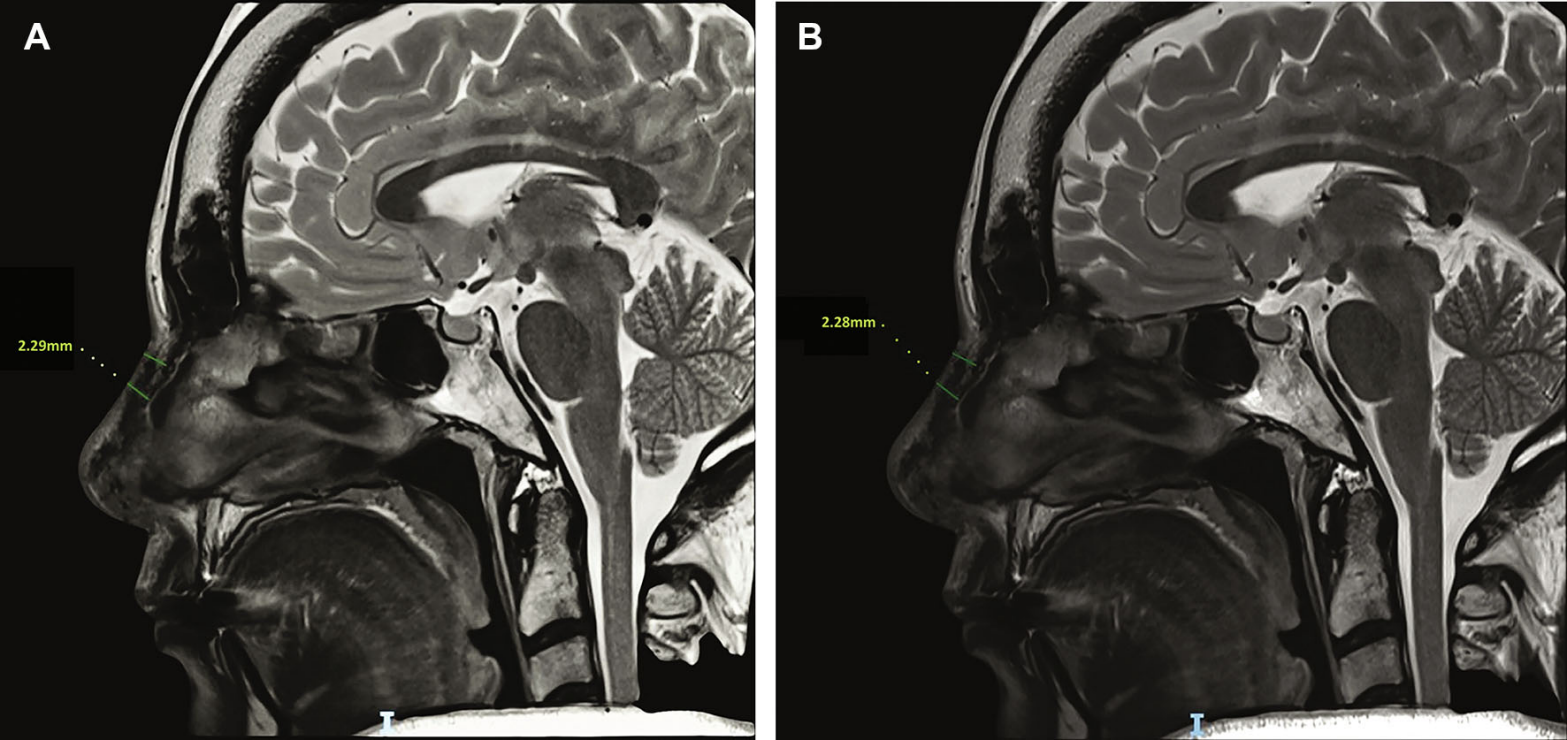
Example of a 2-week postoperative MRI a. The fascia construct maintains its volume and shape throughout the postoperative period and as confirmed by the 18 months postoperative MRI b, no major graft resorption occurred in our series
